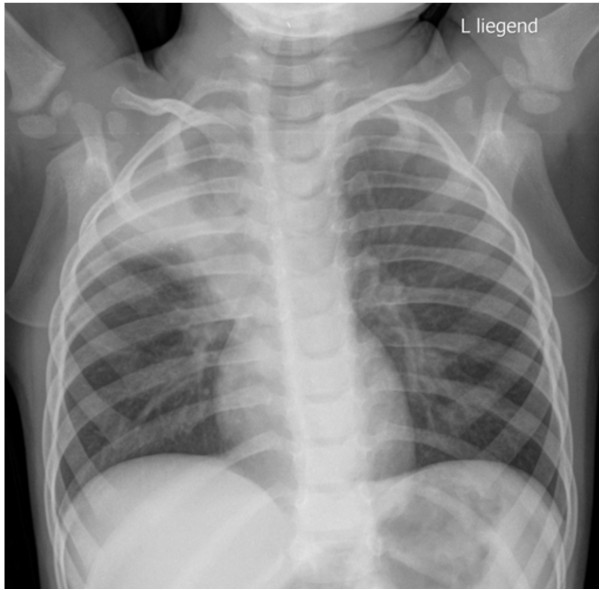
Chest X-ray of case 2 taken on admission with partial atelectasis of the right upper lobe with distinct signs of volume loss of the right lung.
